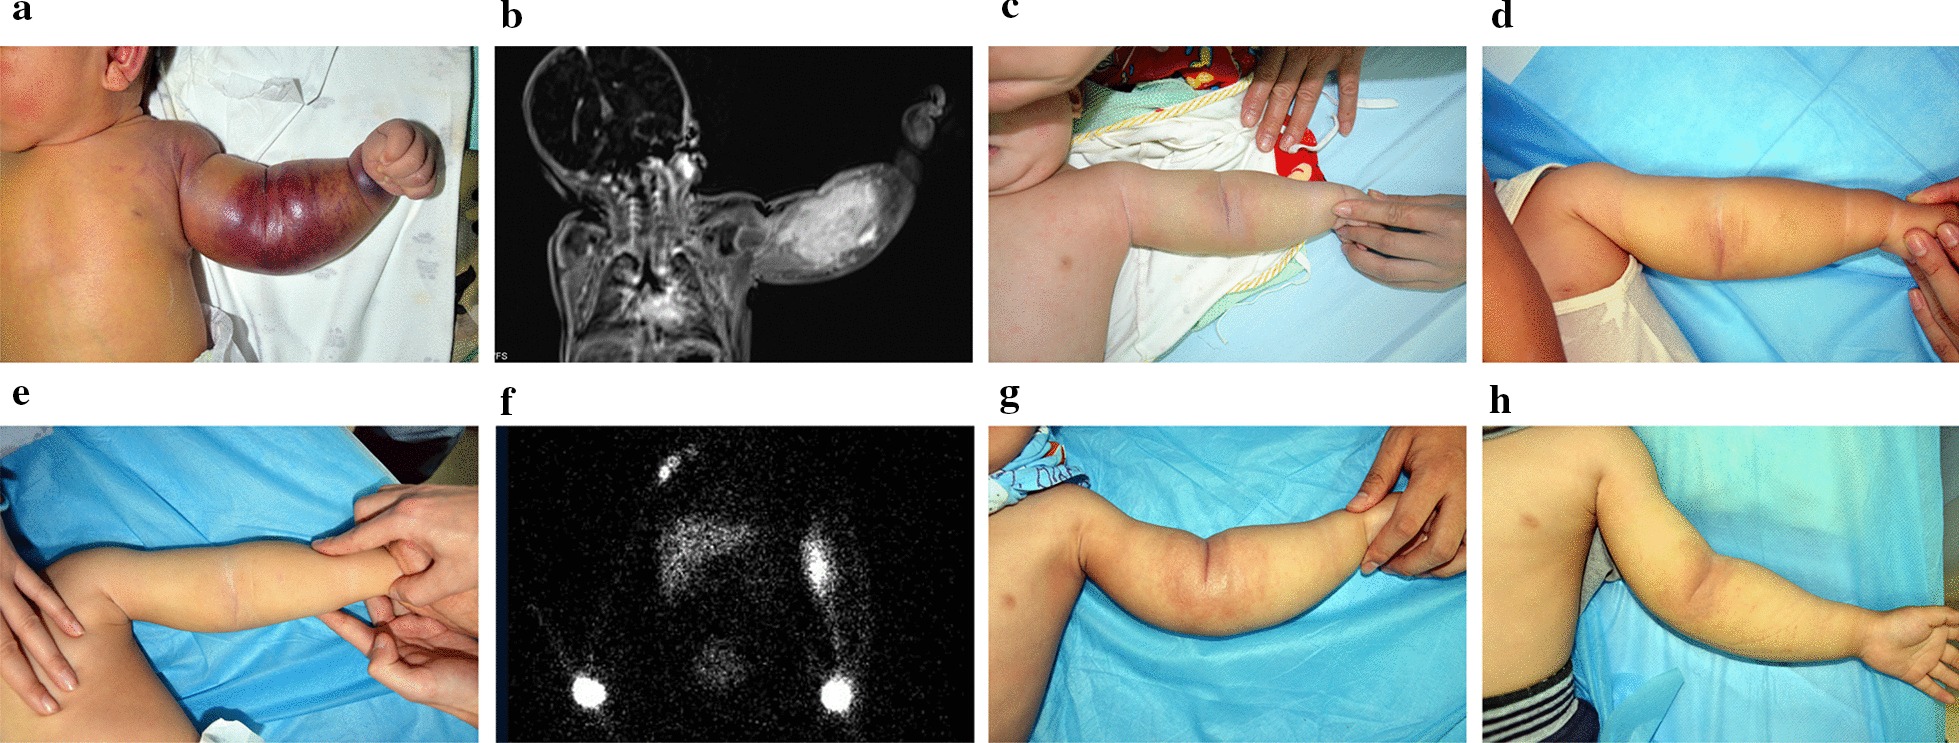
Development of chronic lymphedema in a male with congenital KHE with KMP. a A 2-day-old male infant was referred due to a congenital vascular mass on his left upper extremity. The mass was indurate and purpuric. His platelet count was 9 × 10 [9]/L. b Coronal T2-weighted MRI revealed a hyperintense left upper extremity mass with ill-defined margins and reticular stranding in multiple tissue planes. Photographs at 6 (c), 12 (d) and 18 (e) months after sirolimus treatment showed a decrease in extremity swelling. f At 2 years of age, lymphoscintigraphy demonstrated the absence of draining tracts and axillary node drainage 2 h after injection, with backflow in cutaneous collateral lymphatics. A diagnosis of lymphedema was made. The sirolimus therapy was tapered and discontinued. g Three months after the cessation of sirolimus; the photograph showed a rebound of the extremity swelling, requiring sirolimus reintroduction. h At 3.5 years of age, the patient still received a low dose of sirolimus (trough level, 3–5 ng/ml)
